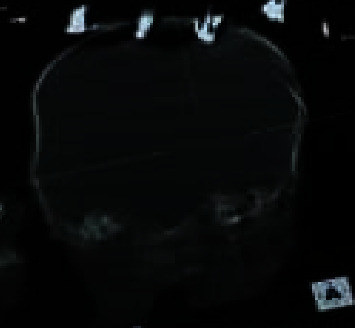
Bone window head CT scan in coronal section showing 2 hyperdensities from either side the midline with metal artifact.
